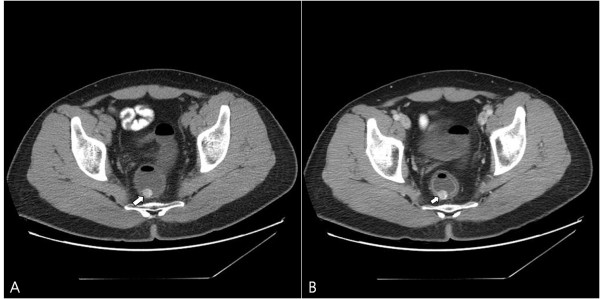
Plain (A) and enhanced (B) computed tomography of the abdomen reveals a prominent contrast-enhancing rectal wall mass with internal calcification (arrow).
